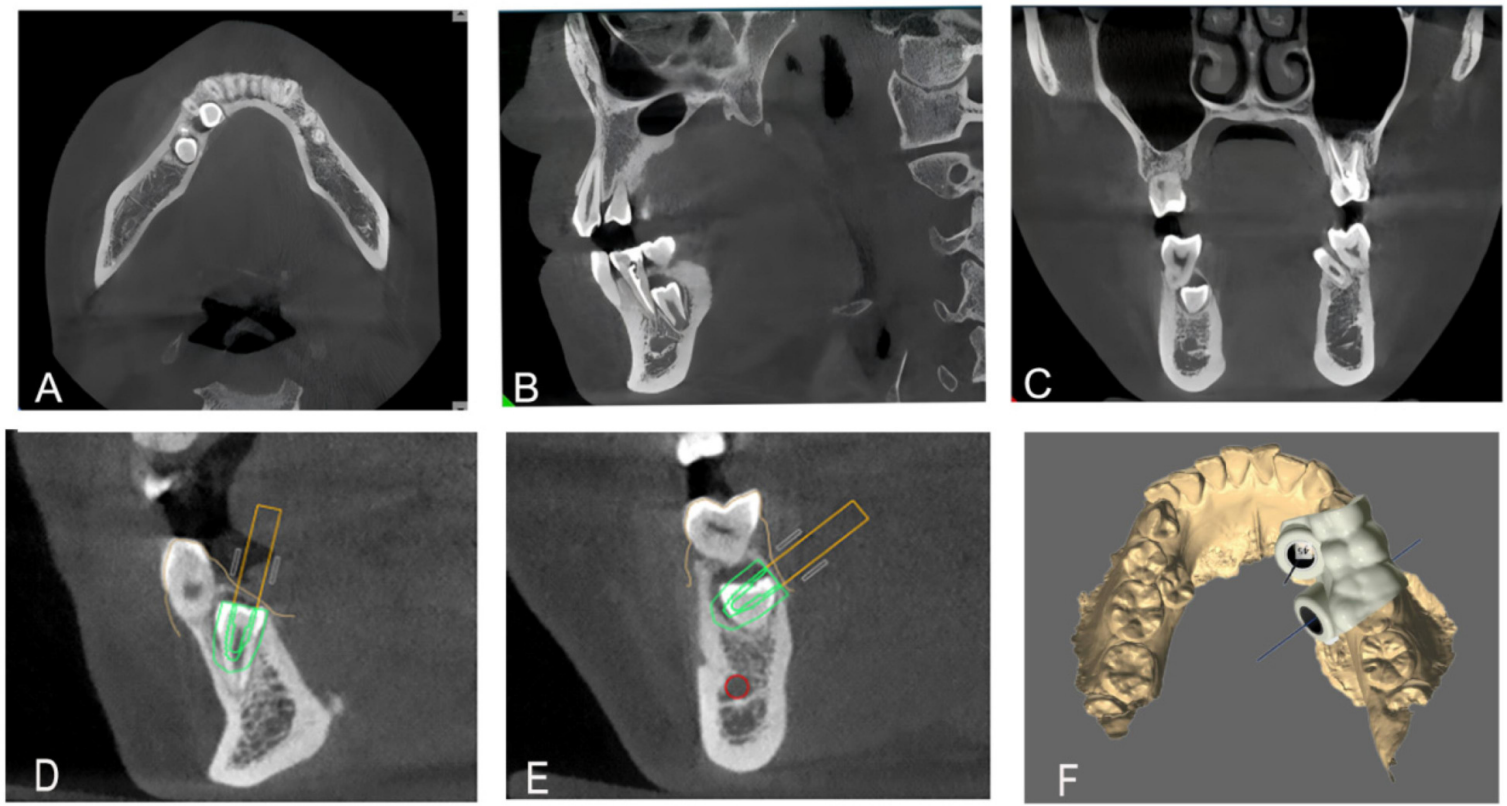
Preoperative CBCT examination revealed the position of the supernumerary teeth in the axial (A), sagittal (B), and coronal planes (C), (D–F). Digital 3D-printed guide design, guide design.
